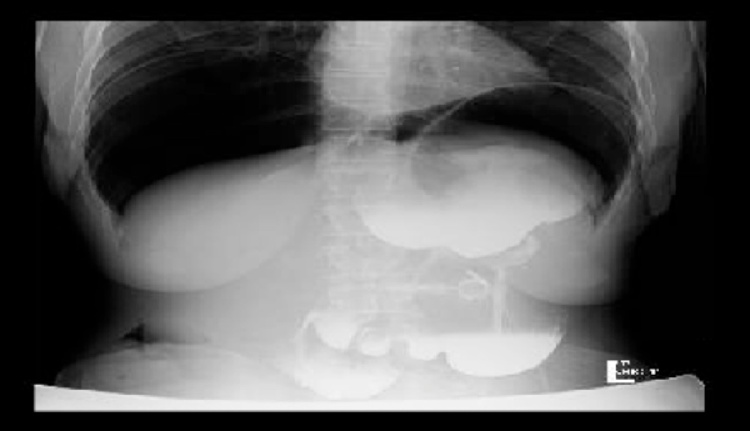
X-ray abdomen (one shout) erect view with oral gastrografin outlined the stomach and duodenum with huge dilated funds and proximal body of stomach proximally to the site of a gastric band which is displaced downward, with no leak.
